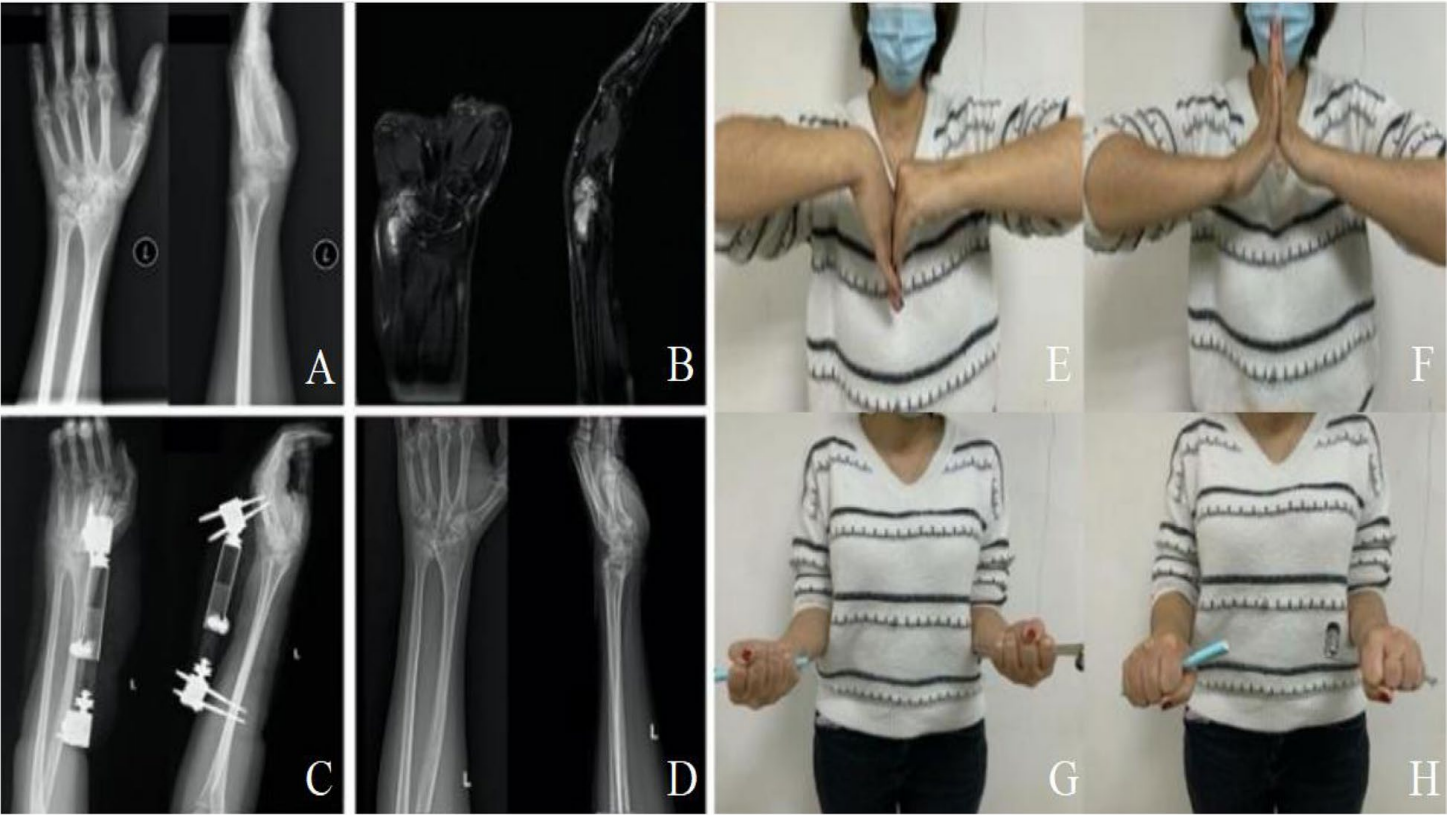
A 43-year-old female patient with Wrist Tuberculosis. A. Anteroposterior and lateral X-rays reveal an osteolytic lesion of the distal radius and the proximal carpal bones. B. MRI images of the left hand showed destructive lesions in the distal ulna and carpal bones, surrounding local effusion, and osteolytic lesions with stenosis of the intercarpal and ulna-carpal joint. C. Anteroposterior and lateral X-rays show 2 days after wrist joint fusion by external fixation. D. The X-ray revealed wrist status 12 months after the removal of external fixation. (E–H). 27 months after the removal of the external fixation, the patient's left wrist function recovered well
